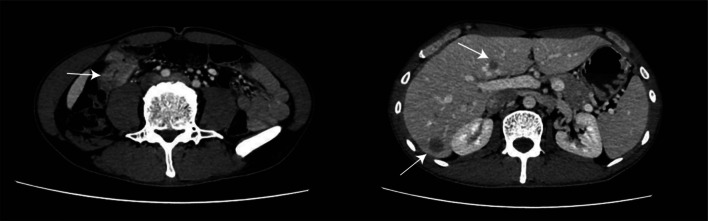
Representative CT-scan images of Case 1. In the hepatic area of the colorectal region, the presence of an occupation raises suspicion of colonic carcinoma. There were a few observed lymph-node metastases nearby, along with intrahepatic nodular shadows, suggesting the possibility of metastatic tumors
